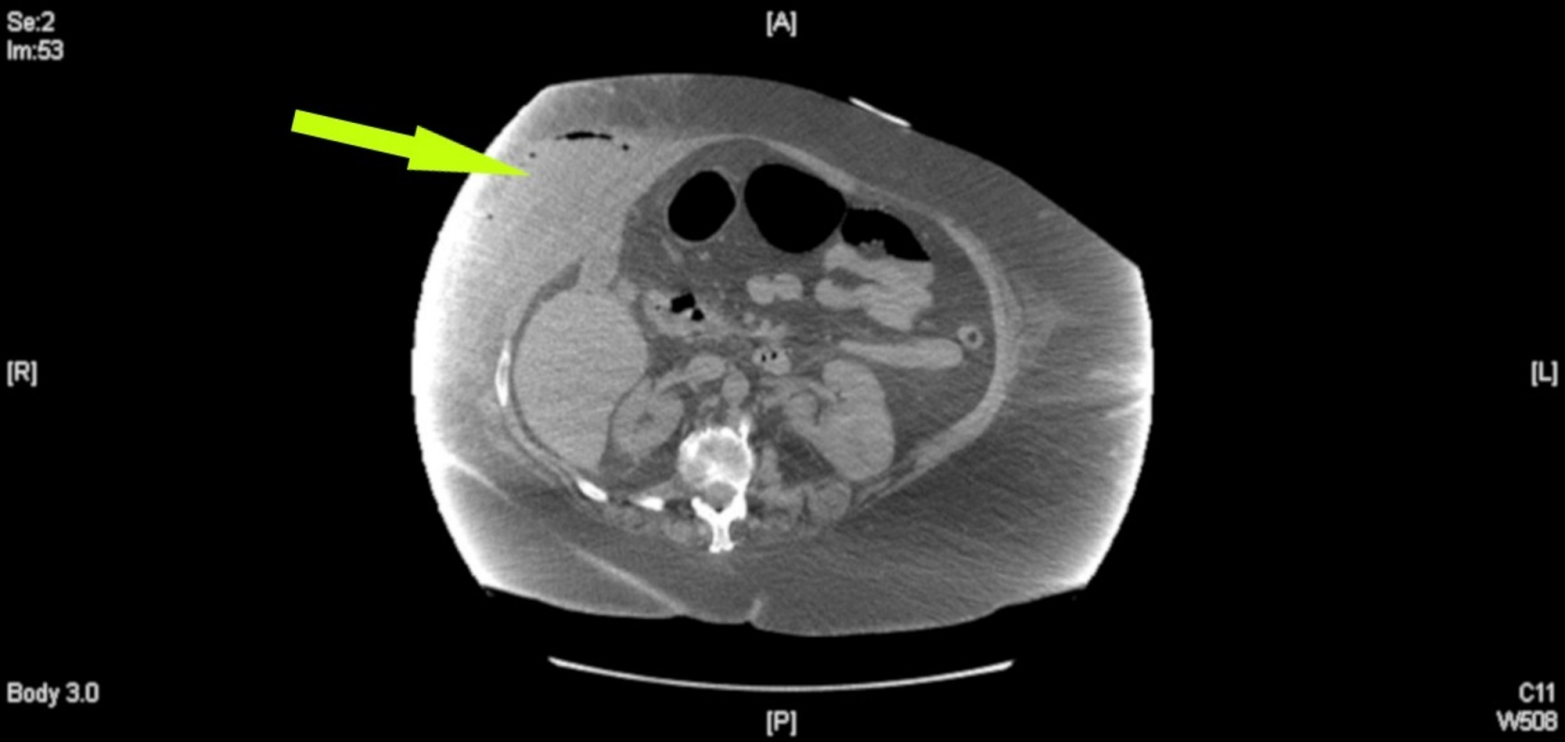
Additional axial view of noncontrast computed tomography of abdomen. Arrow shows large subcutaneous fluid collection noted over right upper quadrant with associated fat stranding and air-fluid levels.
